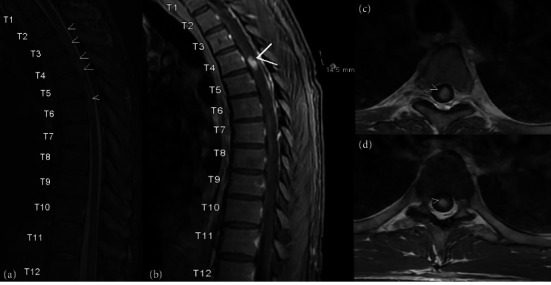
MRI T-spine with and without contrast. (a) T2 sagittal view with hyperintensities at multiple levels from T2–T6. (b) Fat suppressed contrast-enhanced T1 sagittal thoracic spine image with hyperintensity at the T4 level. (c-d) T1 postcontrast axial view of T4-T5 with hyperintensity intramedullary showing contrast enhancement.
